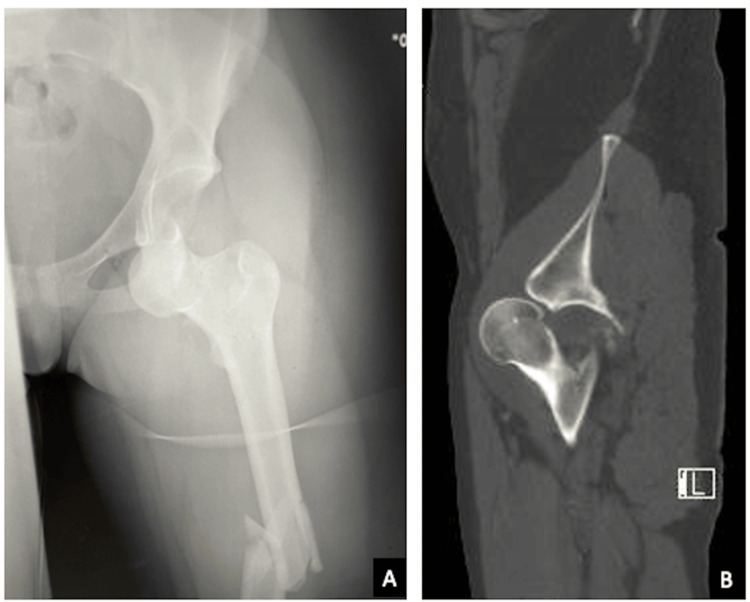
Radiograph of an obturator hip dislocation (A). The CT scan of the same patient reveals intra-articular fragments resulting from a concomitant fracture of the anterior wall of the acetabulum (B).
